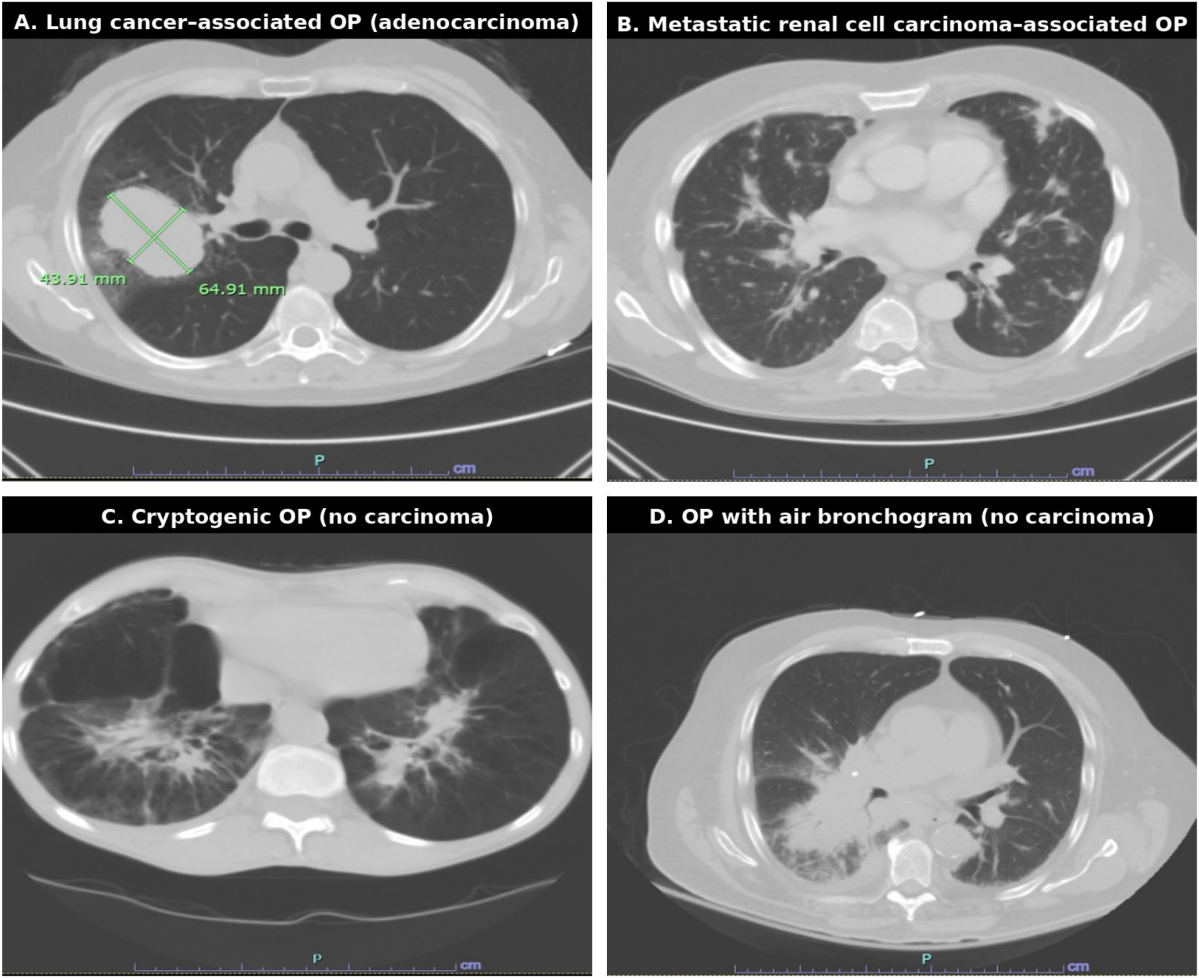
Organizing pneumonia subtypes on CT. Representative CT images of organizing pneumonia (OP): (A) Lung cancer–associated OP (adenocarcinoma). (B) OP associated with metastatic renal cell carcinoma. (C) Cryptogenic OP (no carcinoma). (D) OP with air bronchogram (no carcinoma).
